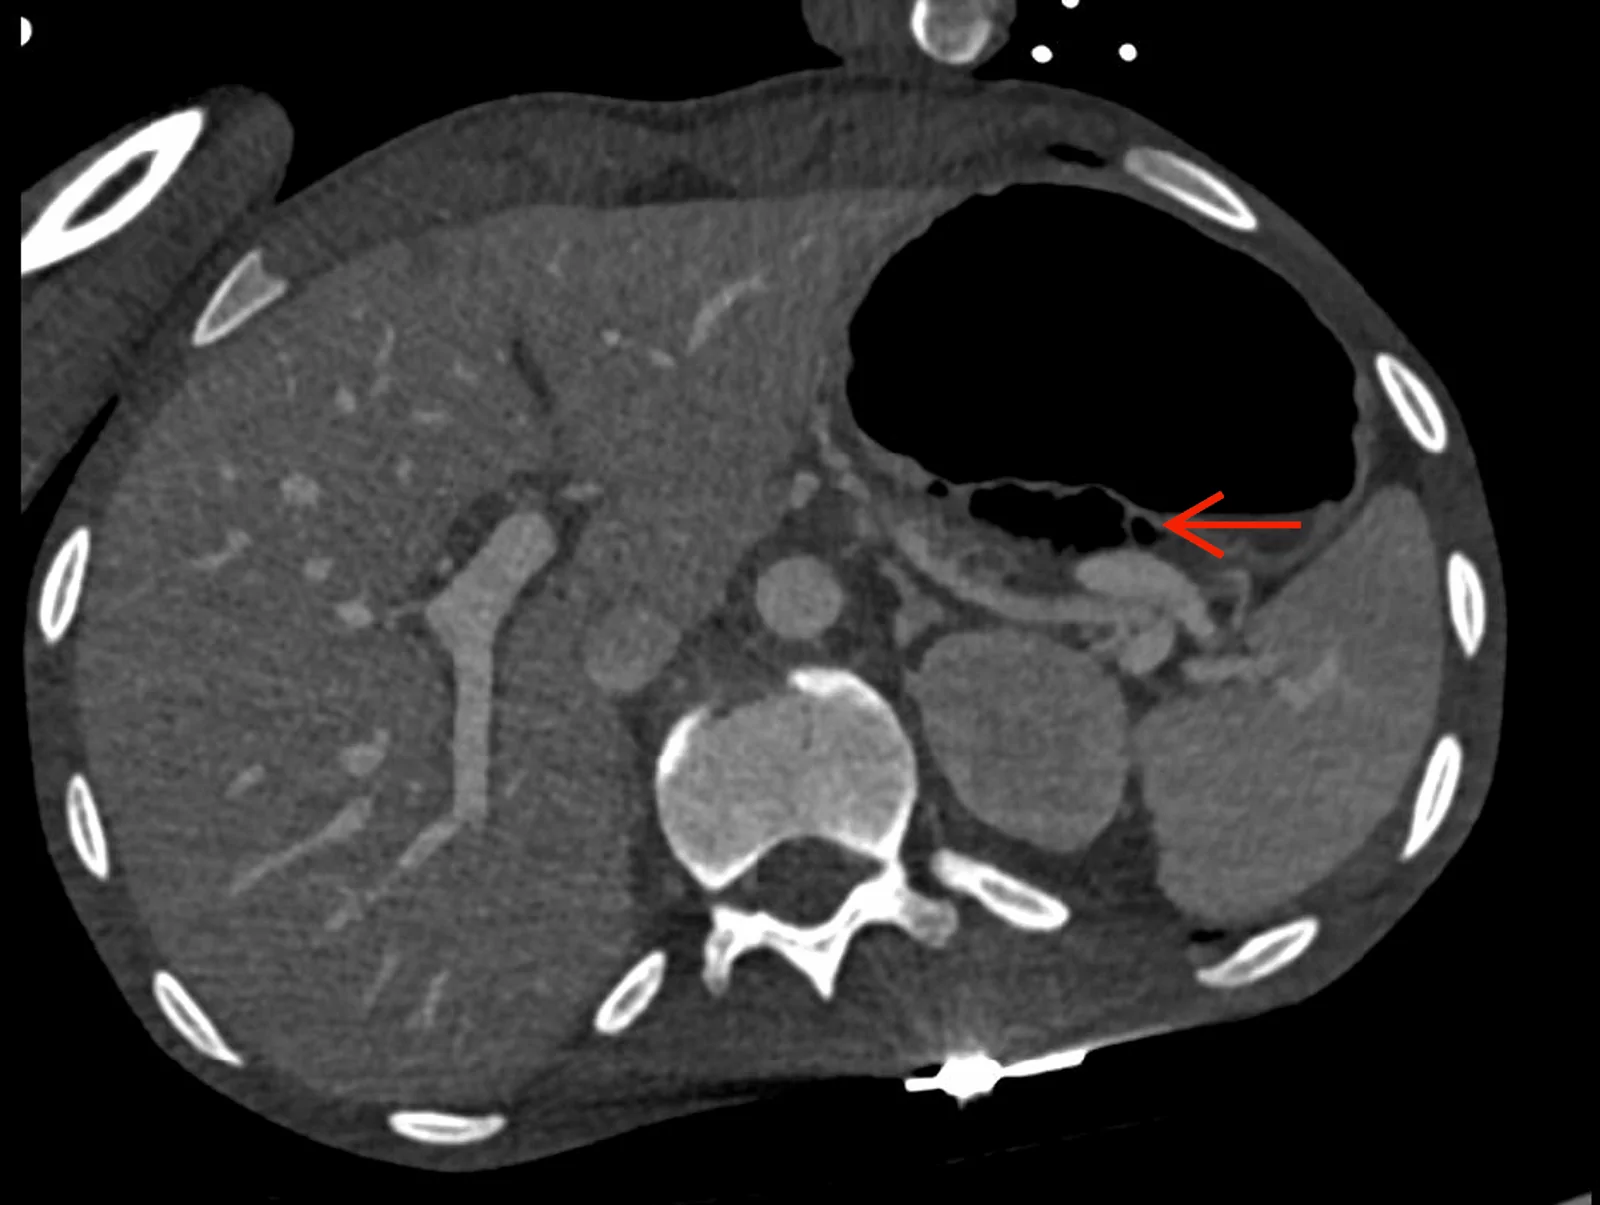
Non-contrast-enhanced CT scan of the abdomen and pelvis demonstrating intramural gas along the gastric wall, consistent with gastric pneumatosis Arrow depicts intramural gas along the gastric wall.
